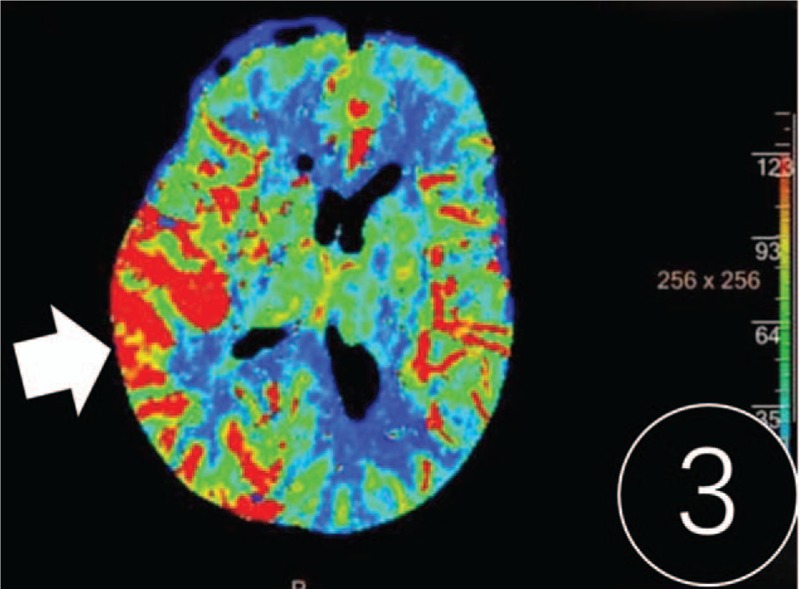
Computed tomography perfusion imaging reflected that the cerebal blood volume of the right temporal occipital lobe was significantly higher than that of the contralateral side.
